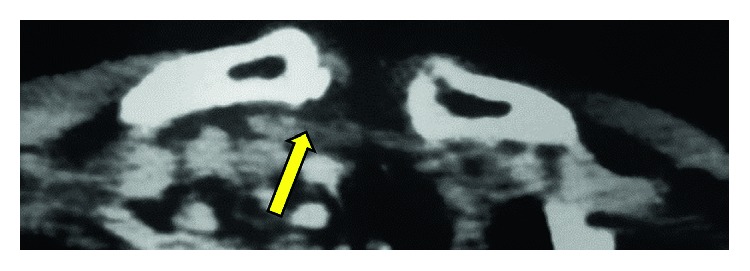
Case 1: a computed tomography scan shows anterior SCJ dislocation (arrow).
